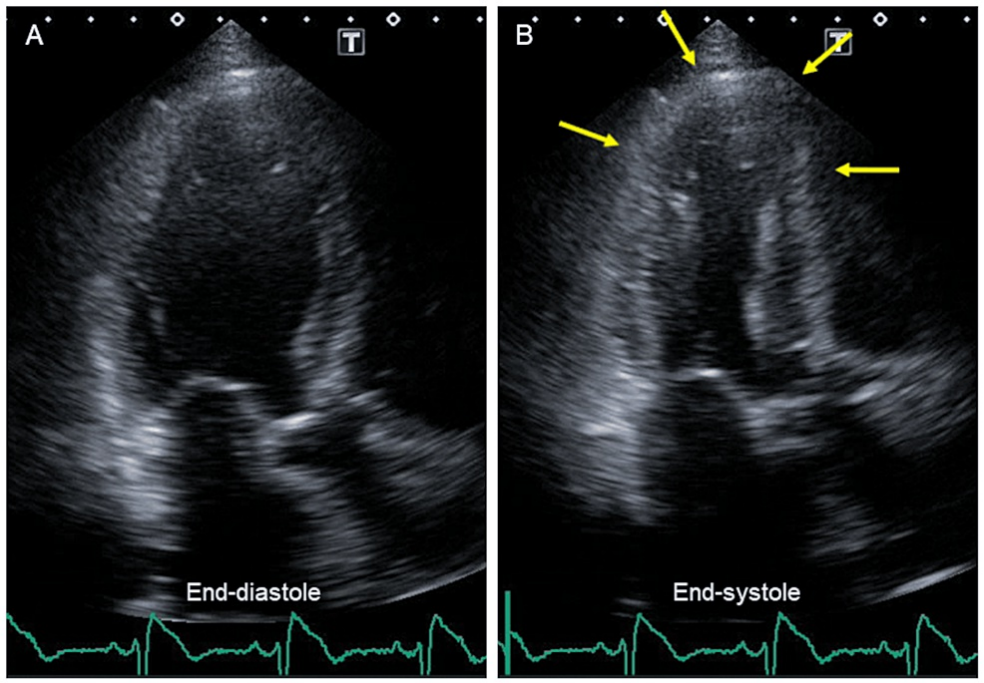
Echocardiogram A TTE showed regional wall motion abnormality in the LV apex with an ejection fraction of 63% (arrows). LV, left ventricular; TTE, transthoracic echocardiogram
